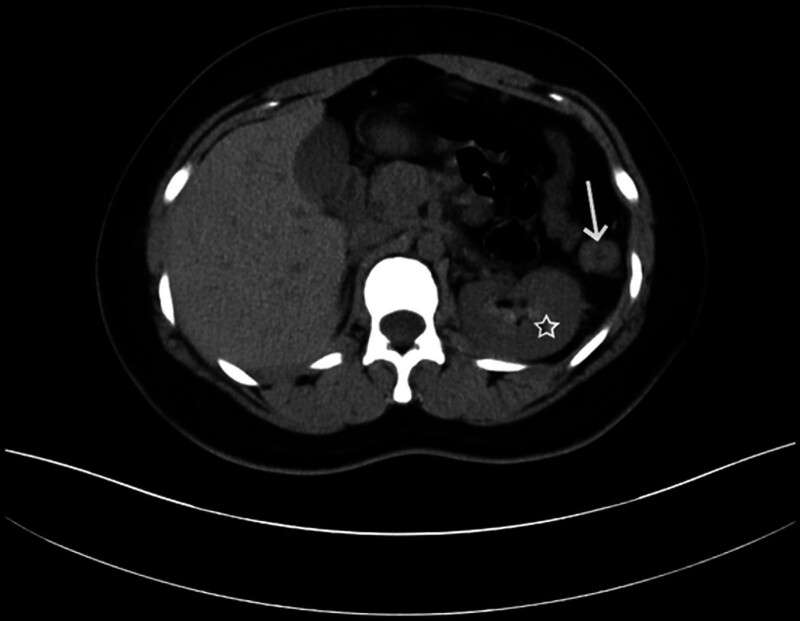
Non-contrast CT showing the lesion in the left upper abdomen ~21 mm × 19 mm × 10 mm (white arrow indicated the lesion and ☆indicated the left kidney). CT = computerized tomography.
